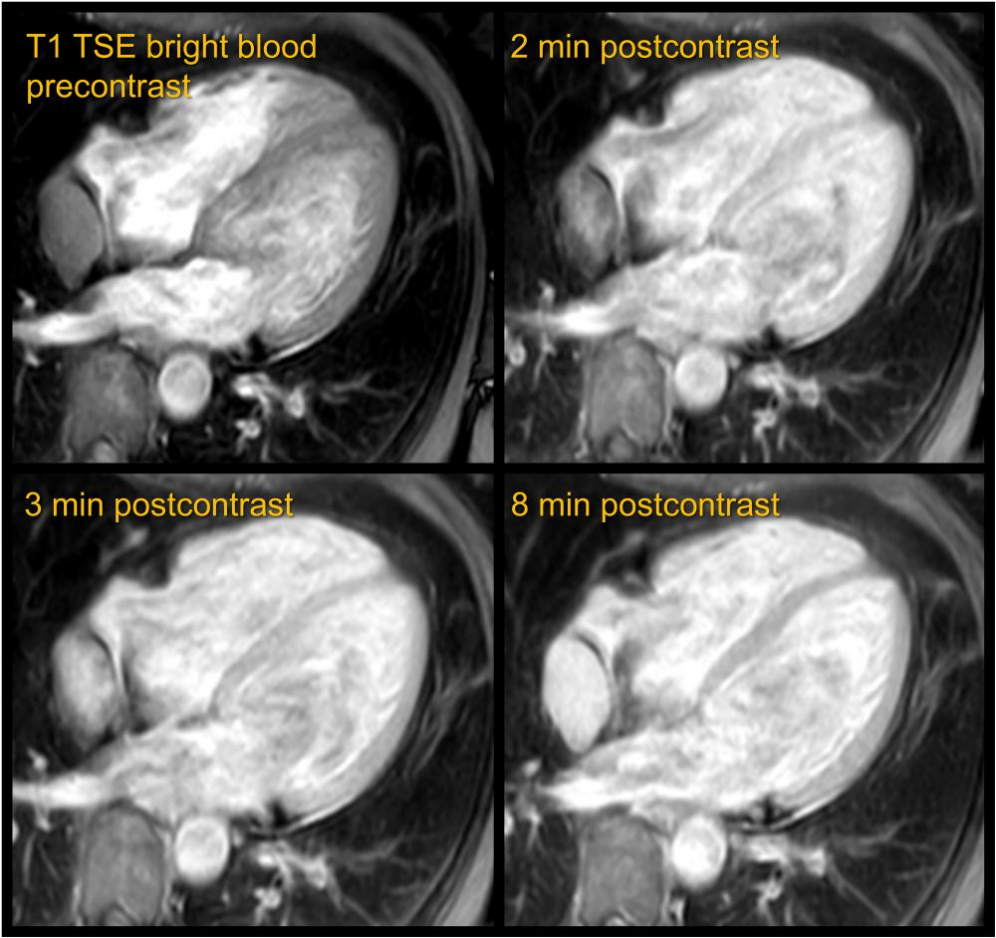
Cardiac magnetic resonance imaging with bright-blood post-contrast imaging. T_1_ turbo-spin-echo bright-blood images were acquired pre-contrast and successively after contrast administration, showing steadily progressing enhancement. Homogeneous enhancement of the tumour, isointense to blood pool, was achieved after 8 min, with persistence at 17 min (not shown). There was no evidence of wash-out, central necrosis, or thrombosis, thus proving the presence of a purely vascular lesion.
